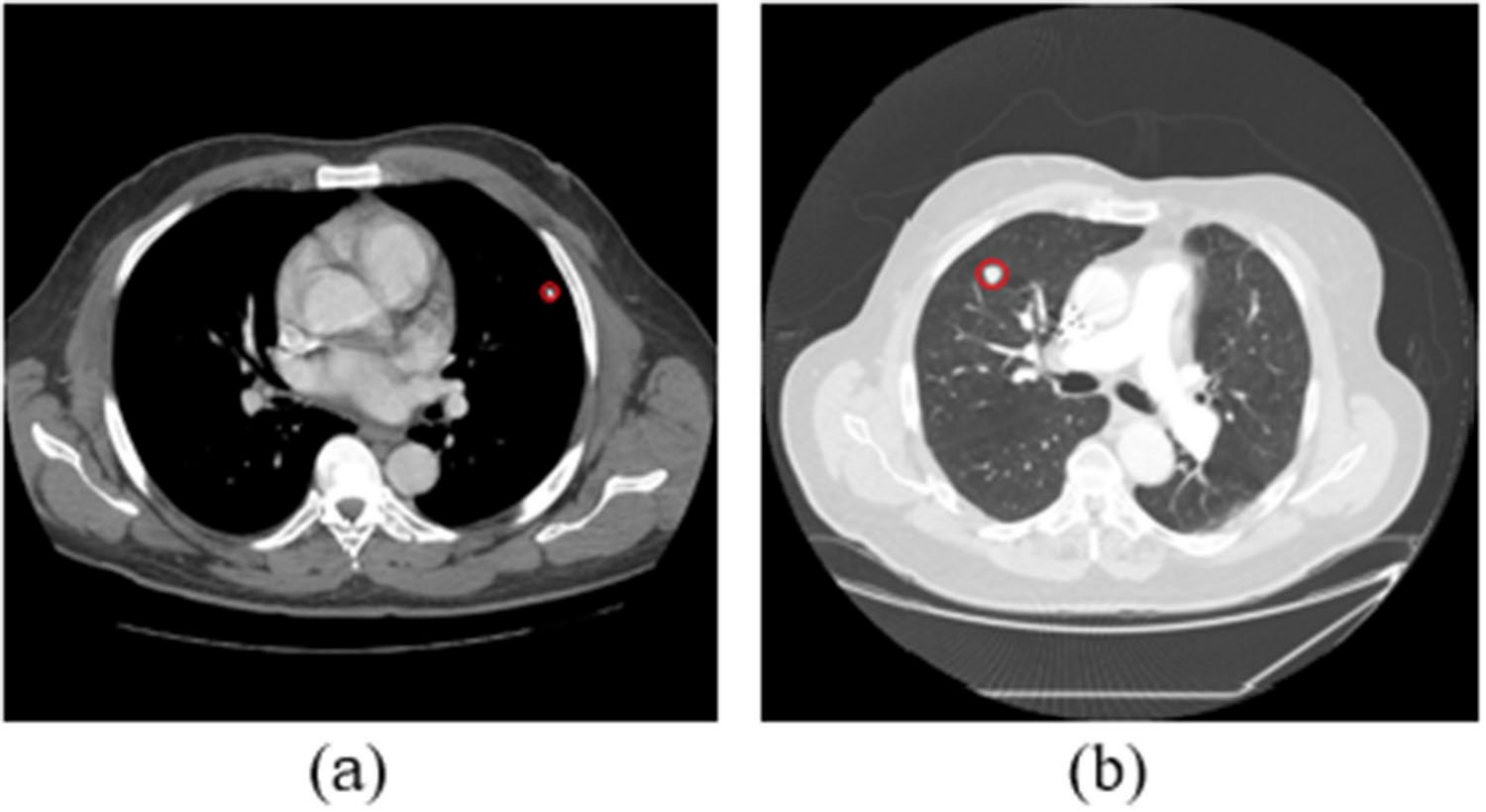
Axial CT images of a Benign, b Malignant pulmonary nodules. Nodules are circled with red color on the images
